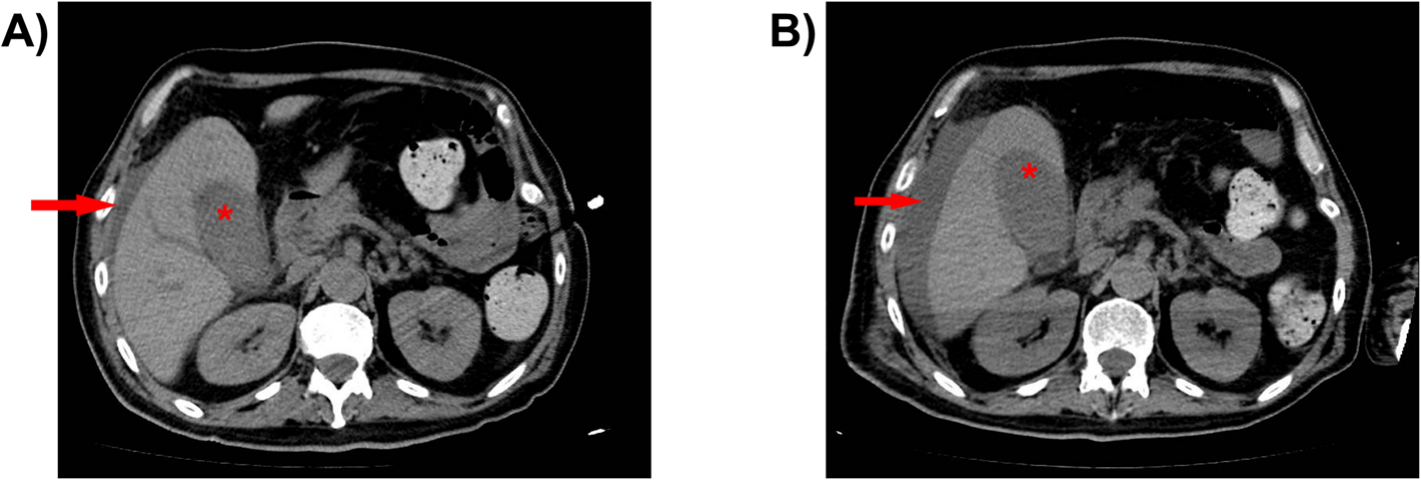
The abdomen CT scan of day 32 (a) and day 33 (b) show relaxed gallbladder with dense content (asterisks) and perihepatic effusion (arrows), which increased in the second CT scan (b)
